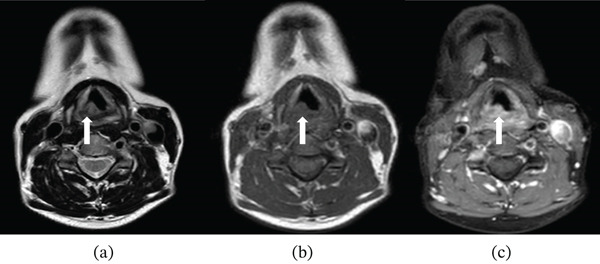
Magnetic resonance imaging of the laryngeal arytenoid neurofibroma. (a) Axial T2‐weighted without fat suppression, (b) axial T1‐weighted without fat suppression, and (c) postcontrast axial T1‐weighted with fat suppression images, with white arrows indicating the lesion.
